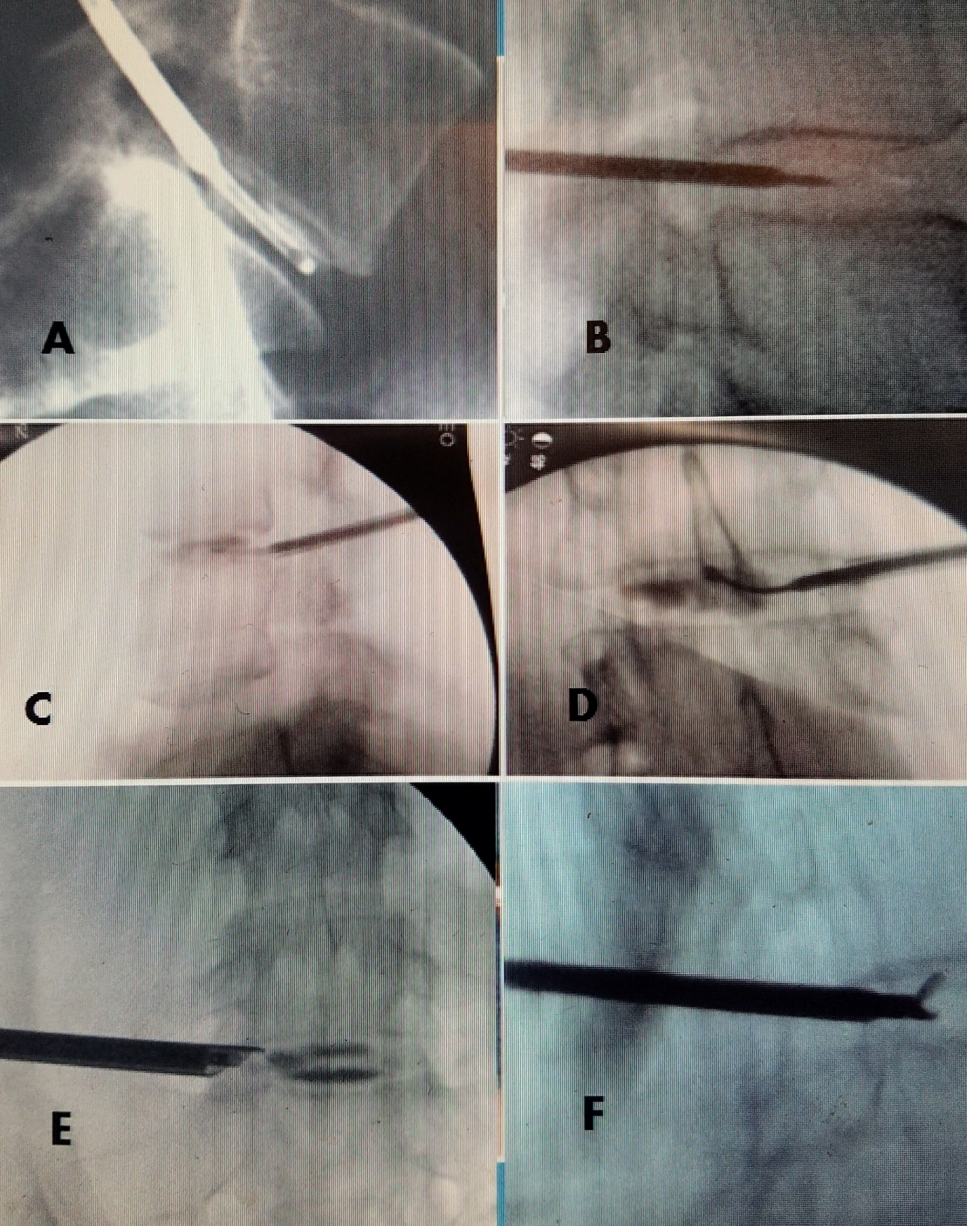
Intra-operative images of different endoscopic and foraminal tubular systems A: 2.1 mm aspirating cutter in the L5-S1 disc space for a contained disc. Note the probe takes up approximately 25% of the disc height and with movement can cover the entire vertical area of the nucleus. B: 2.4 mm straight cannula over guide wire into the L4-5 contained herniated disc. Note the size of the cannula relative to the disc height. C: 1.9 mm cannula placed after a discogram to identify nucleus under fluoroscopy. D: Anterior-posterior view of cannula with intra-discal curved curette crossing the midline within the nucleus. The nucleus is identified and localized with radiographic contrast material in a discogram. E: 6.0 mm lateral beveled transforaminal cannula at margin of L5-S1 after a discogram. Note the dimension of the cannula encompasses most of the vertical height of the disc space allowing easy movement of instruments through the cannula and within the disc. F: L3-4 disc using a 4.8 mm cannula with pituitary ronguer in posterior margin of disc space. The pituitary ronguer is able to move around the disc space making contact with both the superior and inferior extent of the endplates and remove more peripheral disc material.
